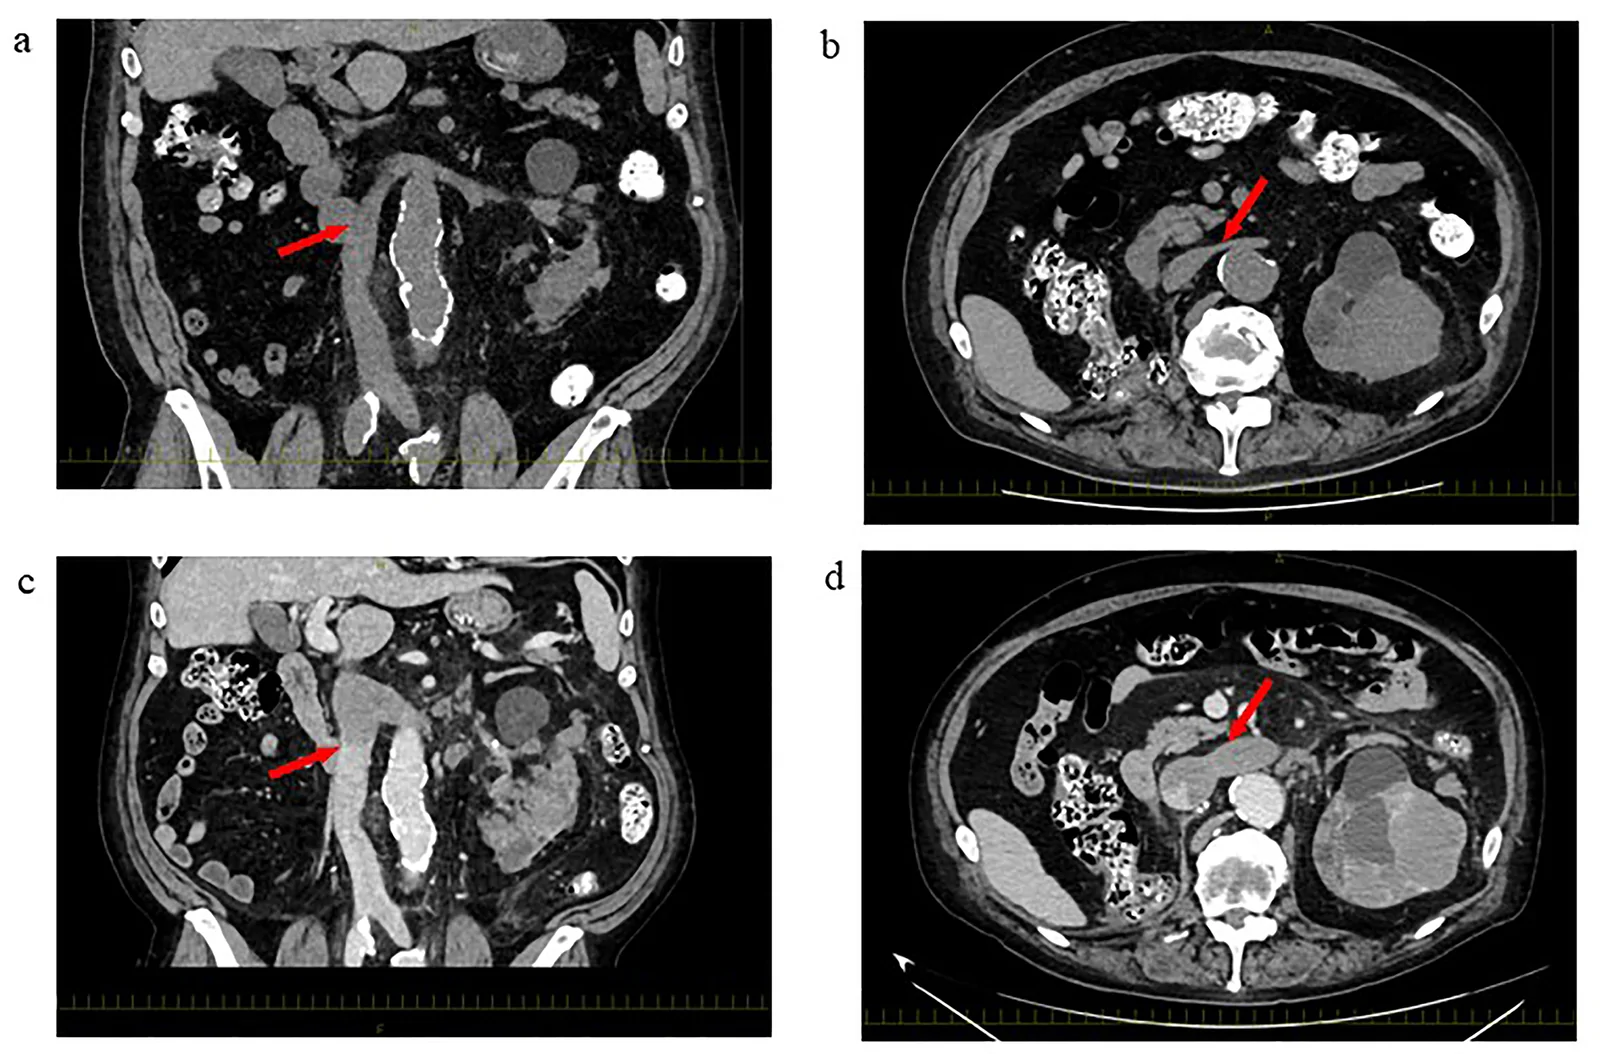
Abdominal CT after radical nephrectomy. (a, c) Inferior vena cava and (b, d) left renal vein. (a, b) No abnormal finding about relapse 4 months after radical nephrectomy. (c, d) Tumor embolisms in the left renal vein 7 months after radical nephrectomy. CT: computed tomography
